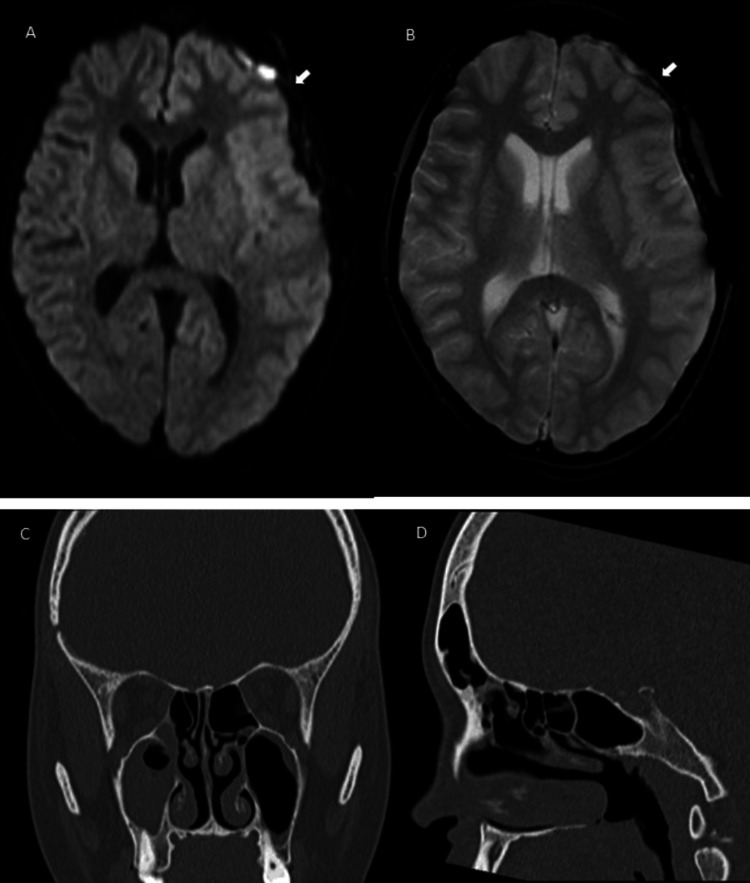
Brain MRI and peri-nasal sinuses CT five days after the last surgery. A - Diffusion-weighted (DWI) magnetic resonance imaging. The arrow indicates residual left frontal subdural empyema; B - Gradient recalled echo (GRE) T2 magnetic resonance imaging. The arrow indicates residual left frontal subdural empyema; C - Coronal plane maxillofacial CT demonstrate decreased filling of the paranasal sinuses; D - Sagittal plane maxillofacial CT. The arrow indicates the left frontal sinus.
